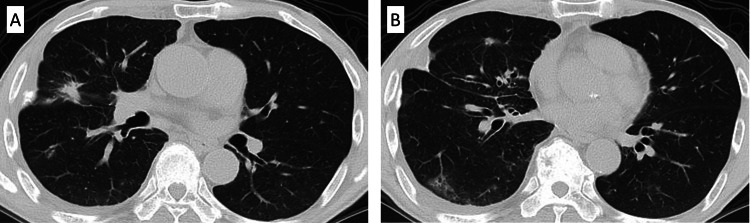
Chest computed tomography after a month since the initiation of steroid treatment Chest computed tomography after the initiation of steroid treatment showed improvements of solid lesions (A) and the pleural effusion, and the disappearance of the ground-glass shadows in both lungs (B).
